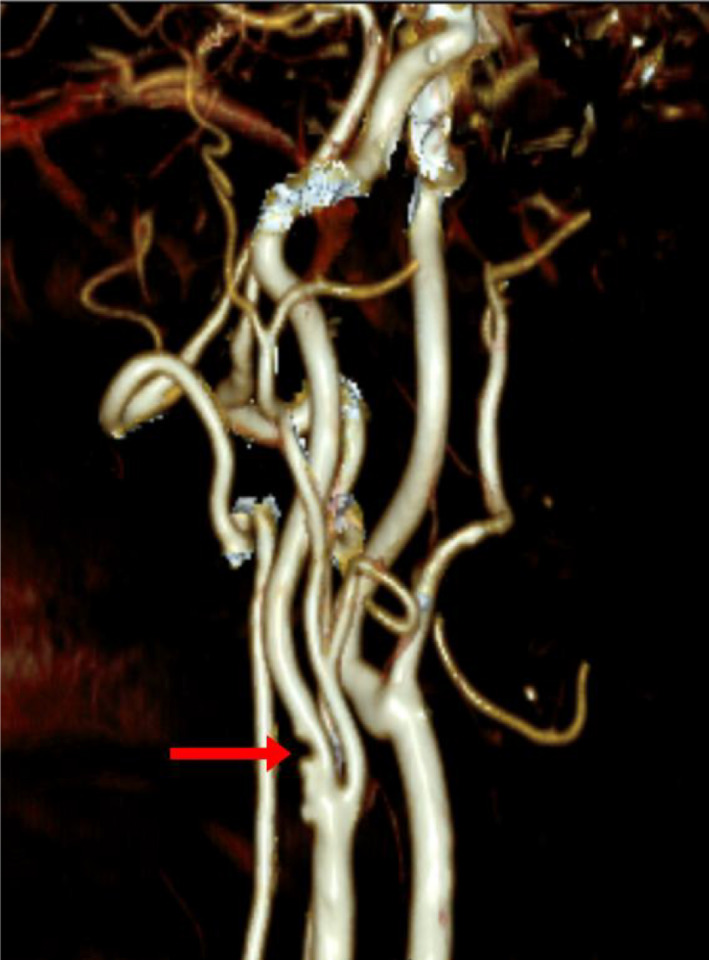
3D reconstructed CTA showing irregularity of the posterior wall of proximal right ICA
